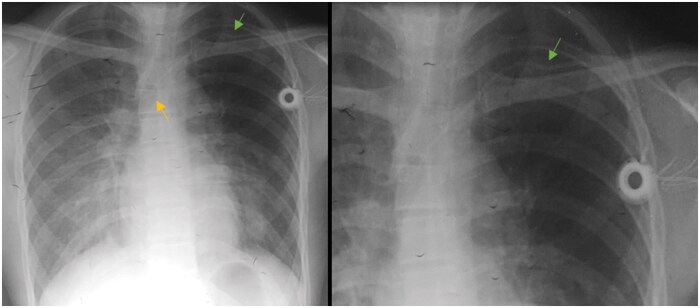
(A) Chest X-ray performed to control the position of the central catheter in the left subclavian vein with the tip ending in the superior vena cava (yellow arrow). (B) Catheter was compressed at thoracic inlet between first rib and clavicle (green arrow), consistent with “pinch-off sign”, grade 1.
